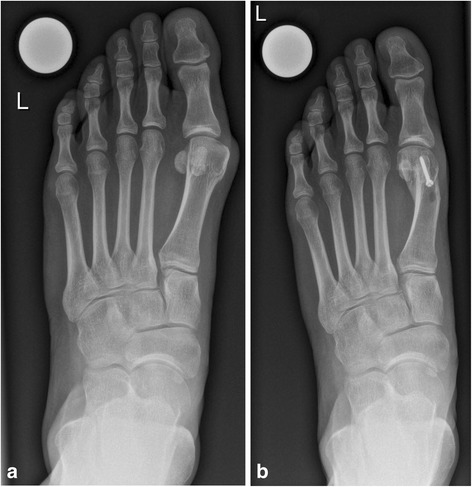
Conventional dorsoplantar radiographs of the left foot in a 37-year-old female. a Preoperative radiograph showing a hallux valgus deformity. b Postoperative radiograph showing correction of a hallux valgus deformity 1 year after reversed L-shaped osteotomy (ReveL) using one screw
